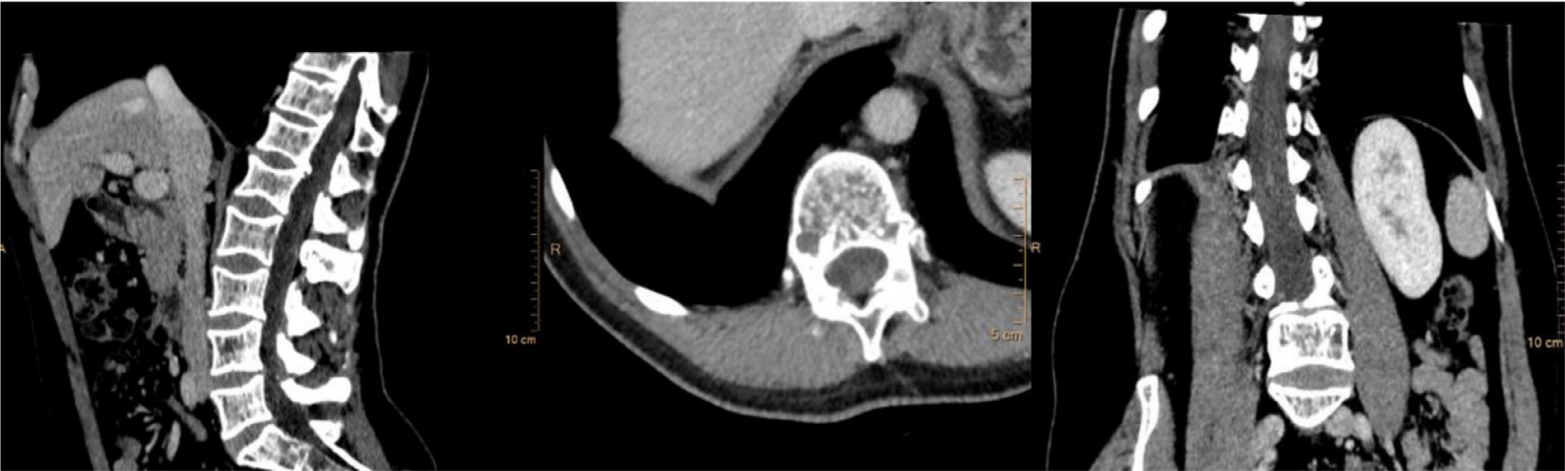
Sagittal, axial, and coronal CT images at the T11-12 level showing the ICM was not visible and no calcifications were present.
